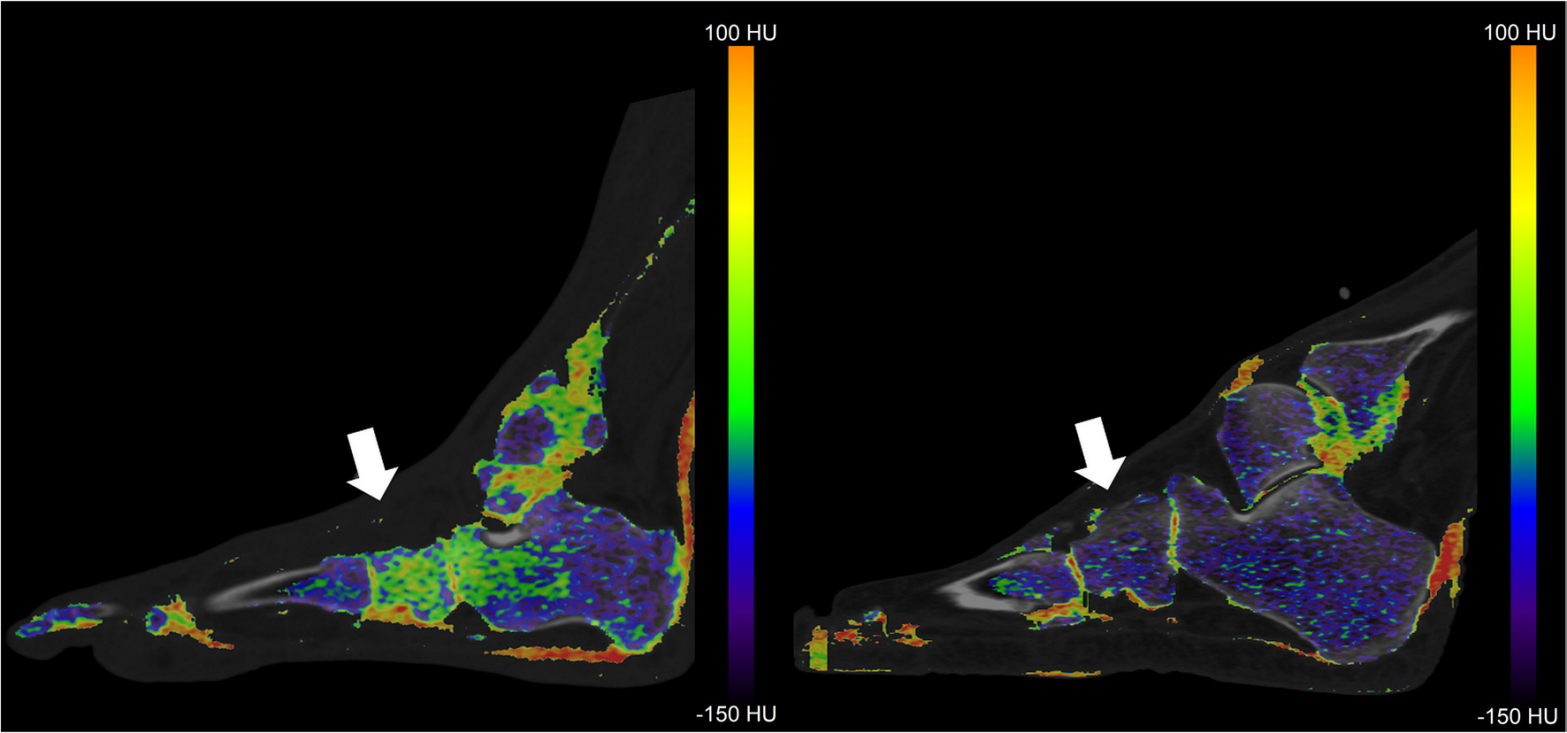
Sagittal VNCa images of a foot with active CN (left) and without active CN (right). The white arrows point to the cuboid bone. In the foot with active CN the average CT value measured in the cuboid bone higher, shown as green or yellow indicating the presence of BME
